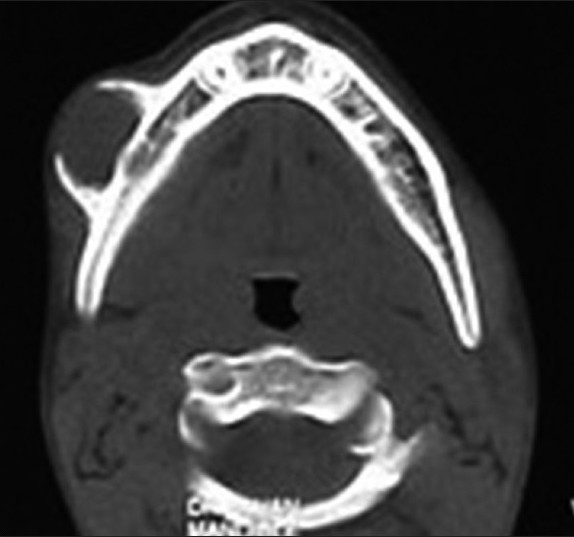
The axial CT view in bone window showing a massive periosteal reaction on the buccal surface, demonstrating the sclerotic borders of the lesion with soft tissue density within the central zone
